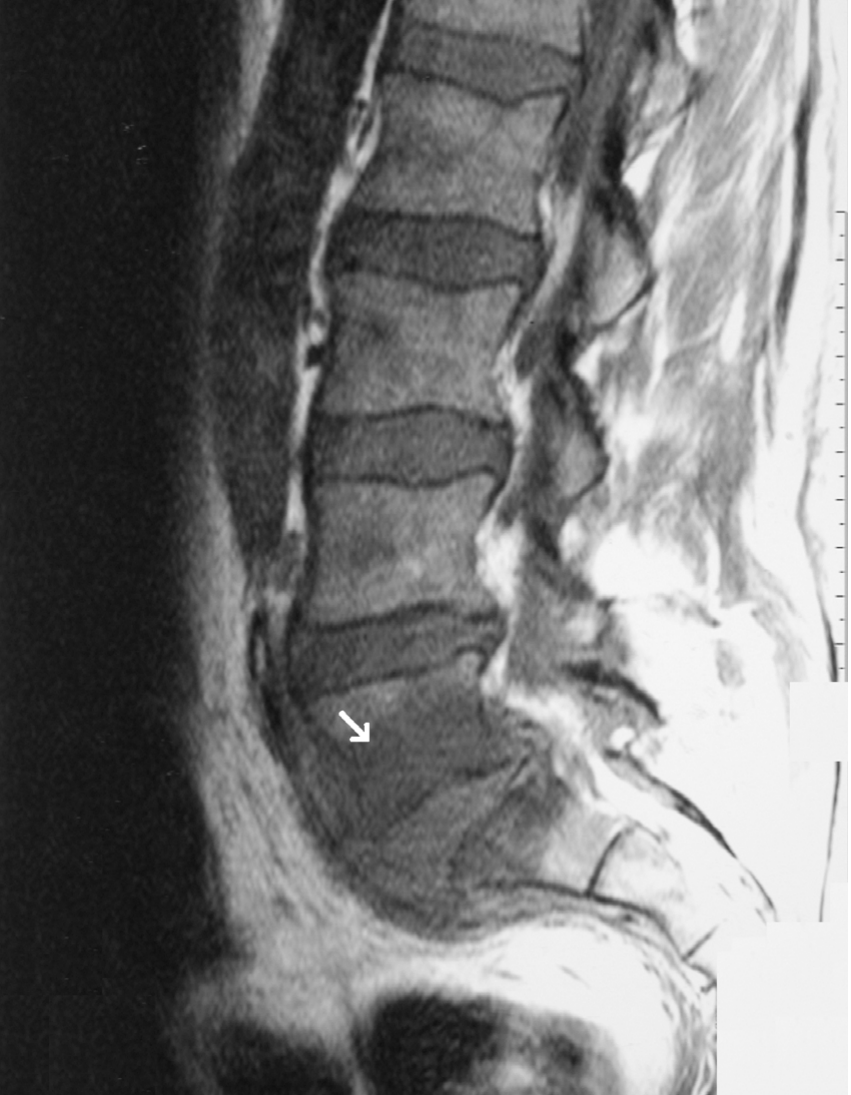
A magnetic resonance imaging scan of the lumbar spine demonstrating abnormal signals of the L5-S1 intervertebral disk. Spinal magnetic resonance imaging revealed diskitis of the L5-S1 intervertebral disk with diffuse edema of the adjacent vertebrae and a paraspinal inflammatory mass with incipient abscess formation.
